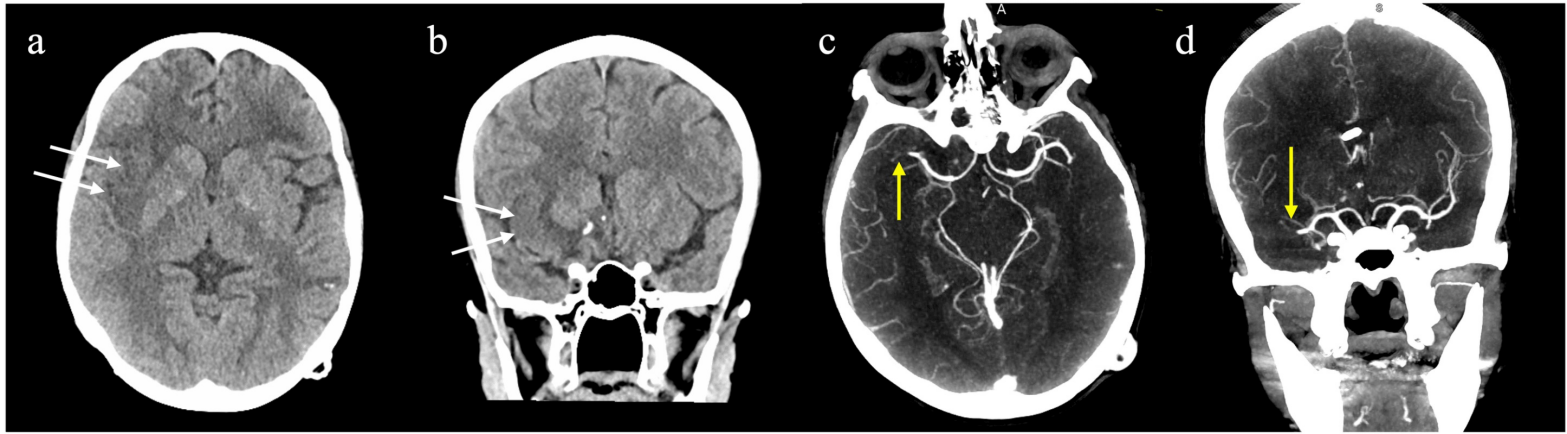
Stroke protocol CT imaging done at the time of presentation demonstrated findings of acute infarction with loss of insular ribbon sign (white arrows) on the unenhanced CT (a,b). The CT angiogram images (c,d) show the right middle cerebral artery (MCA) occlusion with abrupt cutoff of the enhancement (yellow arrows). The left MCA is patent.
